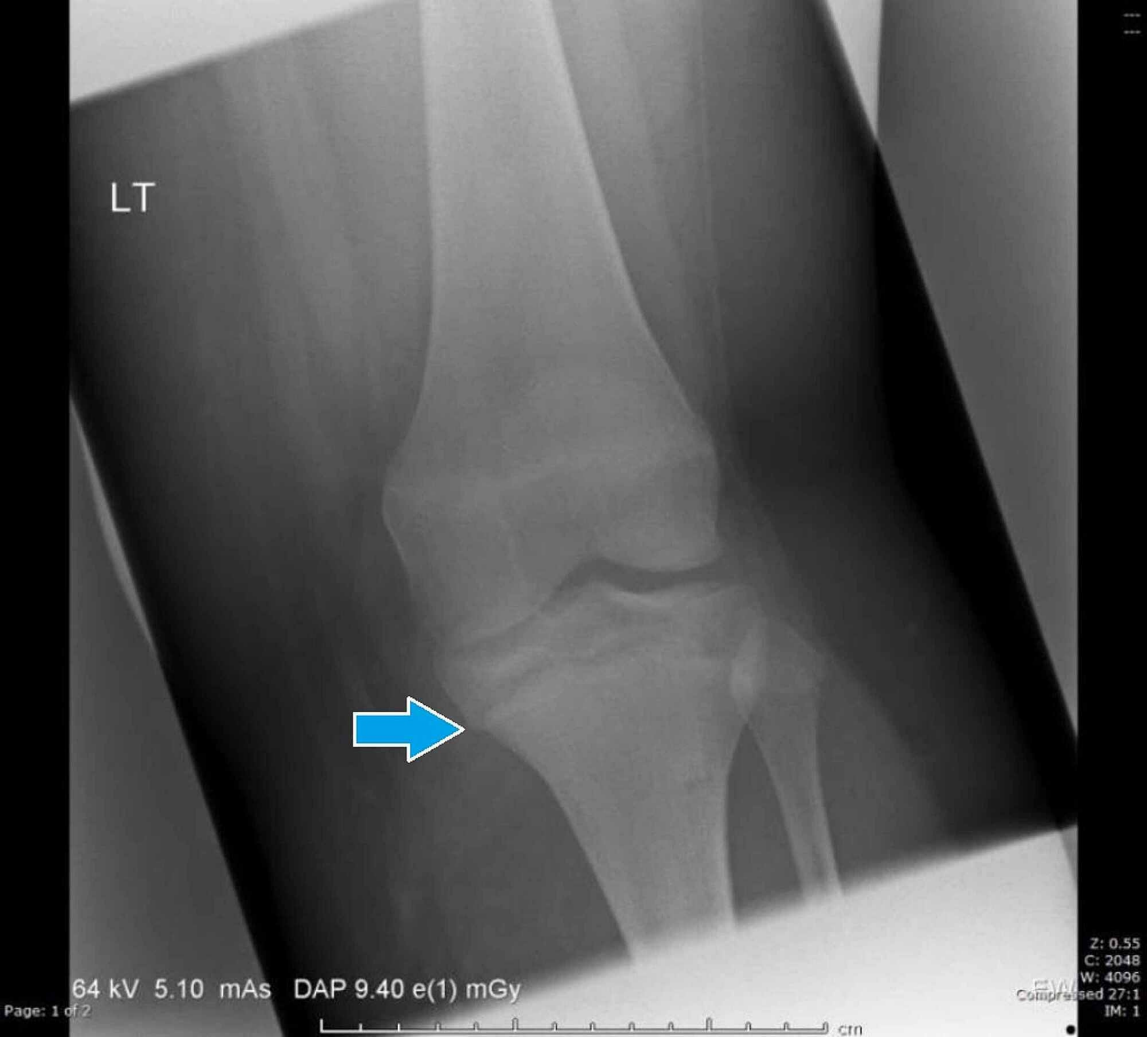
Radiograph of left knee upon initial injury (anterior posterior view)
